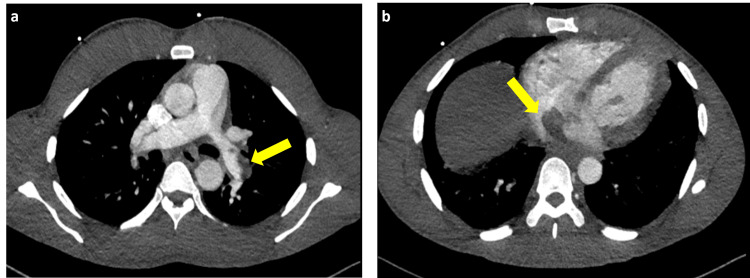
CT Angiogram CT: Computed Tomography; PE: Pulmonary Embolism a) CT Angiogram PE protocol showed partially occlusive thromboemboli within the most distal bilateral main pulmonary arteries extending into upper lobe segmental and subsegmental braches; left main bronchus with the partial filling defect; b) Re-demonstration of filling defect within suprahepatic inferior vena cava with partial protrusion into the right atrium.
